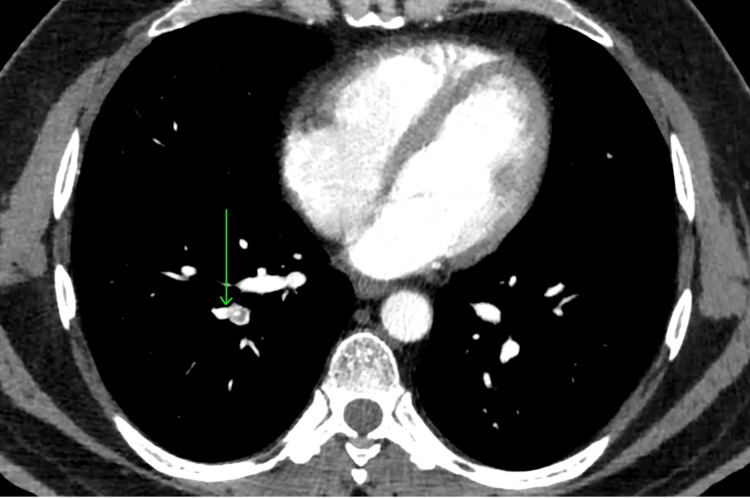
Axial CT pulmonary angiography showing partial hypodense filling defects in segmental and subsegmental branches of the pulmonary arteries, consistent with pulmonary thromboembolism.
